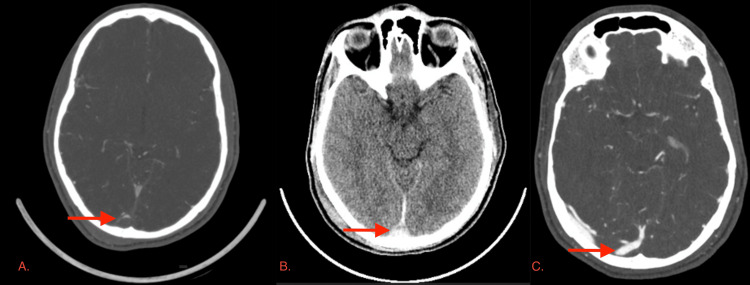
(A) CT cerebral venography showing evidence of thrombus in the torcula. (B) Follow-up CT brain demonstrated persistent hypo-density in the posterior superior sagittal sinus and right transverse sinus. (C) A repeat CTV showing only a small residual filling defect at the torcula, consistent with a small residual thrombus CT: computed tomography, CTV: computed tomography venography
